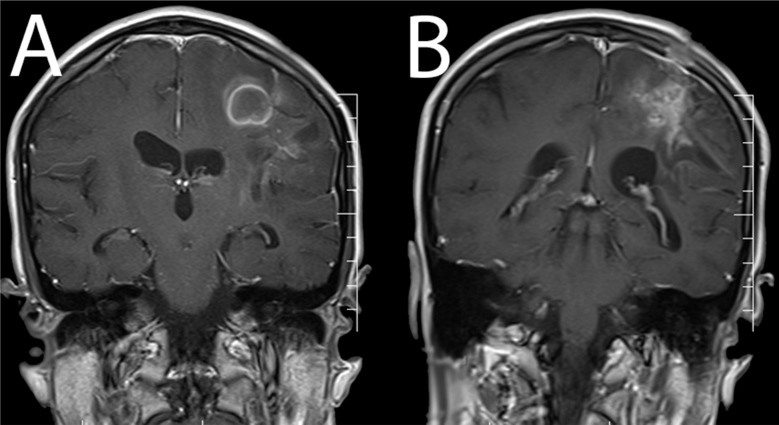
(A) Coronal view of contrast-enhanced magnetic resonance imaging one; (B) 4 months after drainage and antibiotic therapy of the abscesses
